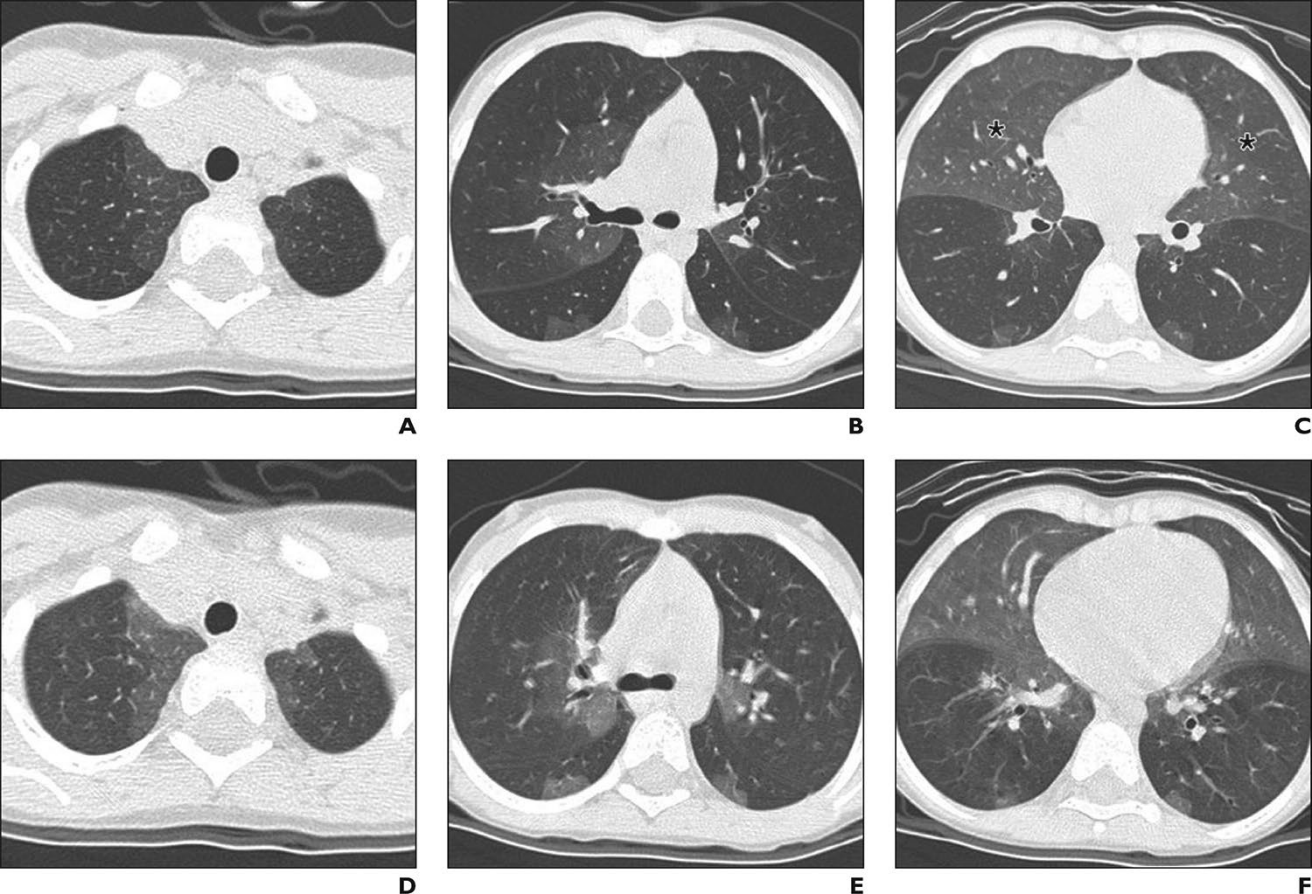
NEHI. A 40-month-old child. A–C Inspiratory CT showed relatively homogeneous GGOs without any structural distortion, particularly in the middle lobe, lingula, and paramediastinal regions. D–F Expiratory CT demonstrated only mild decrease in volume of both opaque and lucent areas, suggesting background air trapping of the entire lung (adapted from AJR 2010, Ref. [28])
